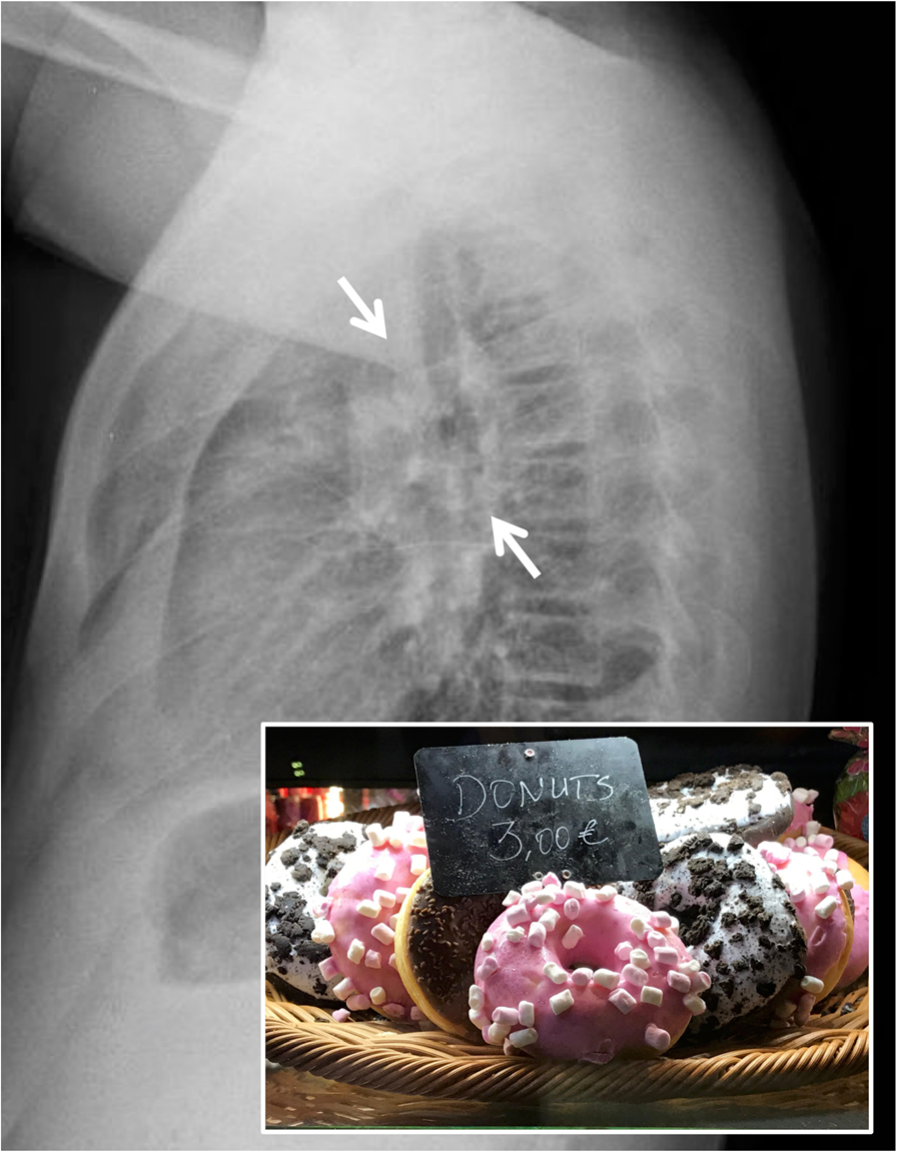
Doughnut sign. A lateral chest radiograph with the “doughnut sign”: the white arrows clearly demonstrate the presence of a white radiopaque ring at hilar region. It is created by enlarged lymph nodes at hilar and subcarinal region, located around intermedius bronchus, and normal profiles of right and left pulmonary arteries and aortic arch anteriorly and superiorly. This radiopaque ring resembles the appearance of a doughnut—as appreciable in the embedded figure. This sign could be suggestive of lymphadenopathy—in case of tuberculosis
